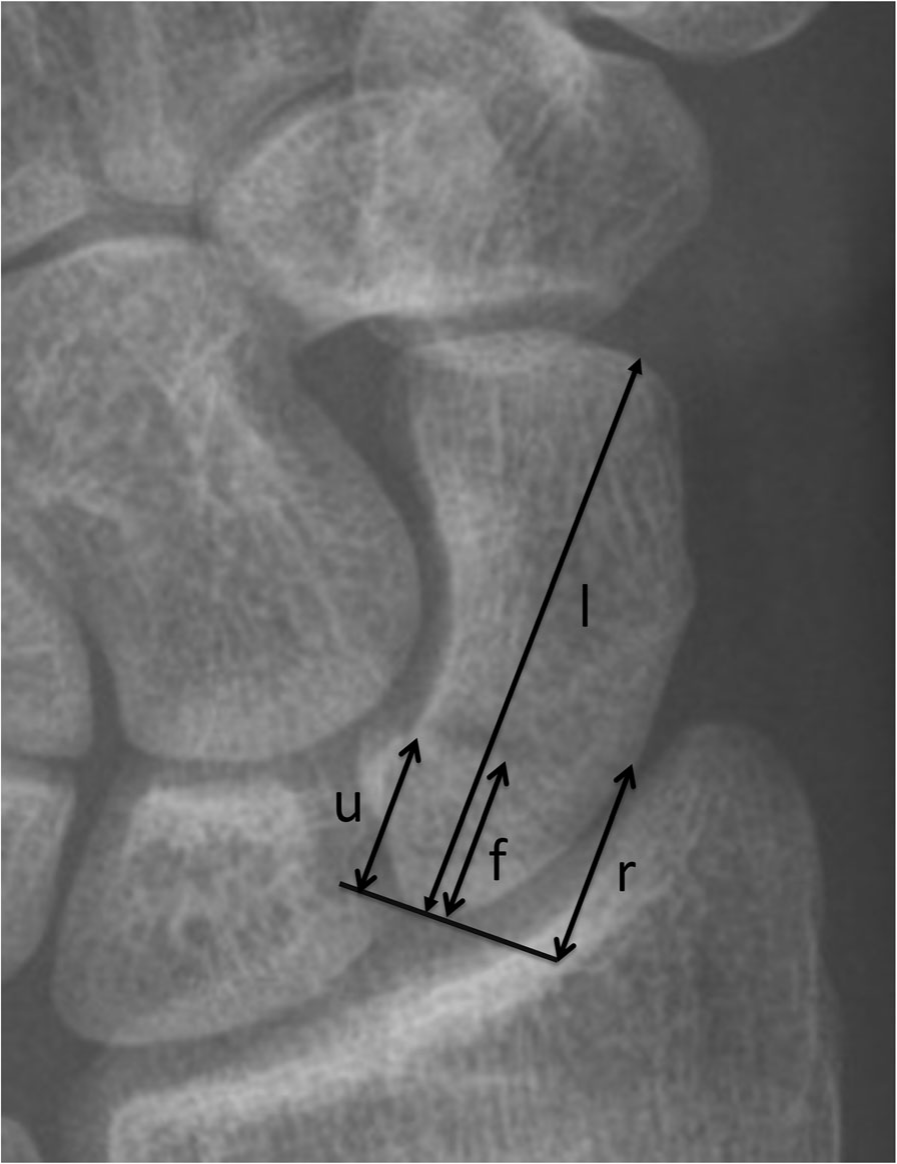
Plain radiograph of a scaphoid fracture demonstrating the measurements made. Detailed legend - the long axis (l) and the distance along the long axis to the fracture site are shown (f, fracture distance), while the ulnar (u) and radial (r) distances to the fracture site are also shown. Note (f) is measured along (l) but for ease of demonstrating the methodology (f) has been moved just adjacent to (l). In this example the long axis measurement is f/l which equals 0.28
